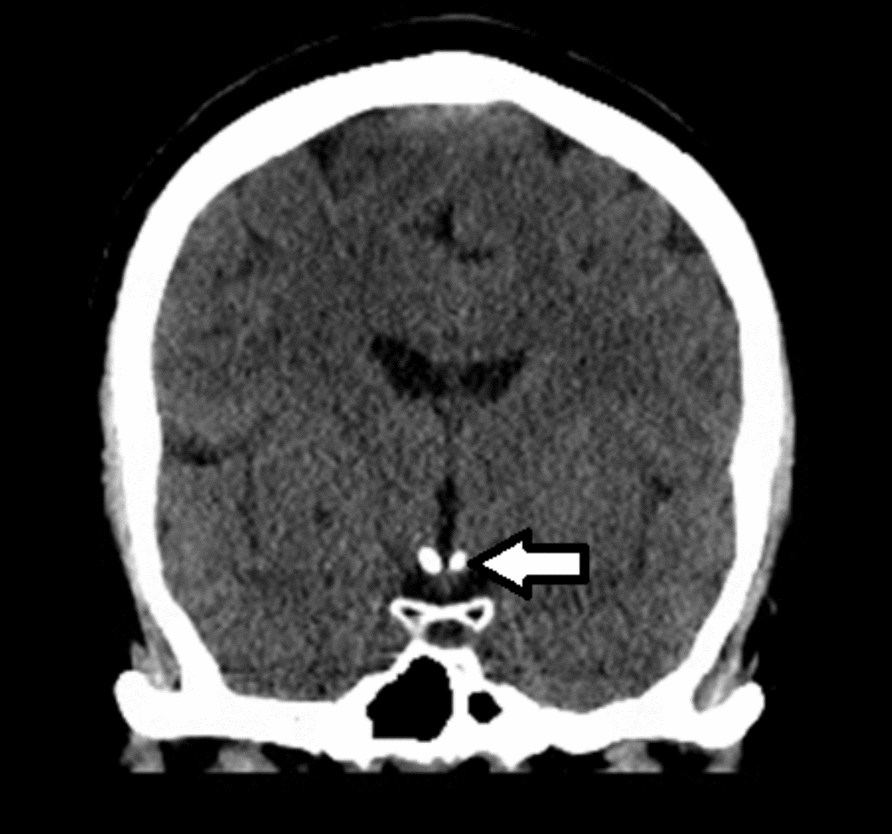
Coronal Computed Tomography (CT) image of the brain demonstrating symmetrical dot-like enhancements in the suprasellar region, characteristic of neurosarcoidosis affecting the pituitary axis
